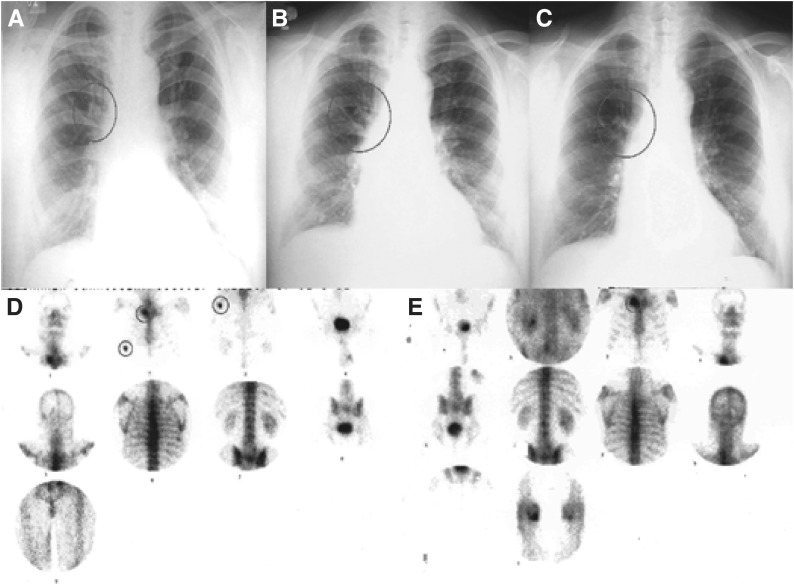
X-ray (A–C) and scintigraphy scans (D, E) showing primary and secondary tumour regression in a 64-year-old patient with NSCLC treated with first-line gefitinib 250 mg day^−1^. At diagnosis in March 2002 (A) and immediately prior to beginning gefitinib (B; July 2002), the patient had a primary tumour in the right upper lobe that partially responded (C; April 2003) for a sustained period, totalling 11 months at the time of reporting. Regression of bone metastases was evident 6 months after beginning gefitinib (D; March 2002, E; January 2003).
